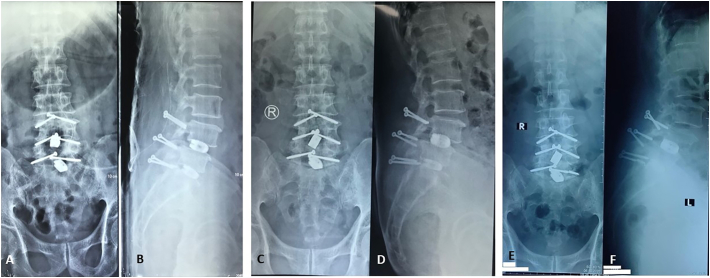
A 56-year-old male with chronic low back pain. Translaminar screw fixation was performed in 3 segments (A, B). X-ray shows progressing spondylolisthesis at L4-L5 (C, D) and broken screw at L5-S1 (E, F).
